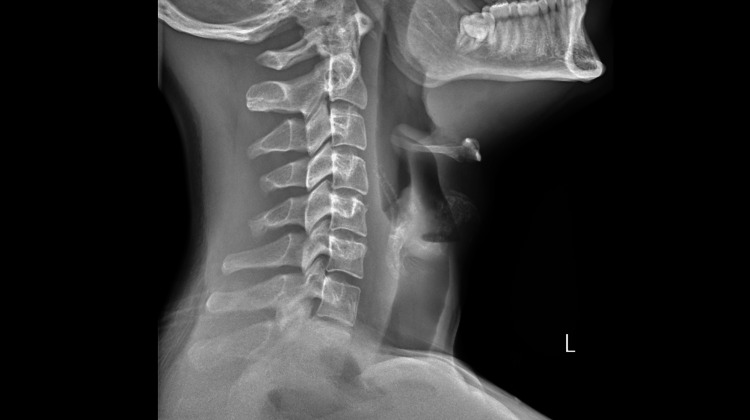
Lateral view neck radiograph showing swollen epiglottis
